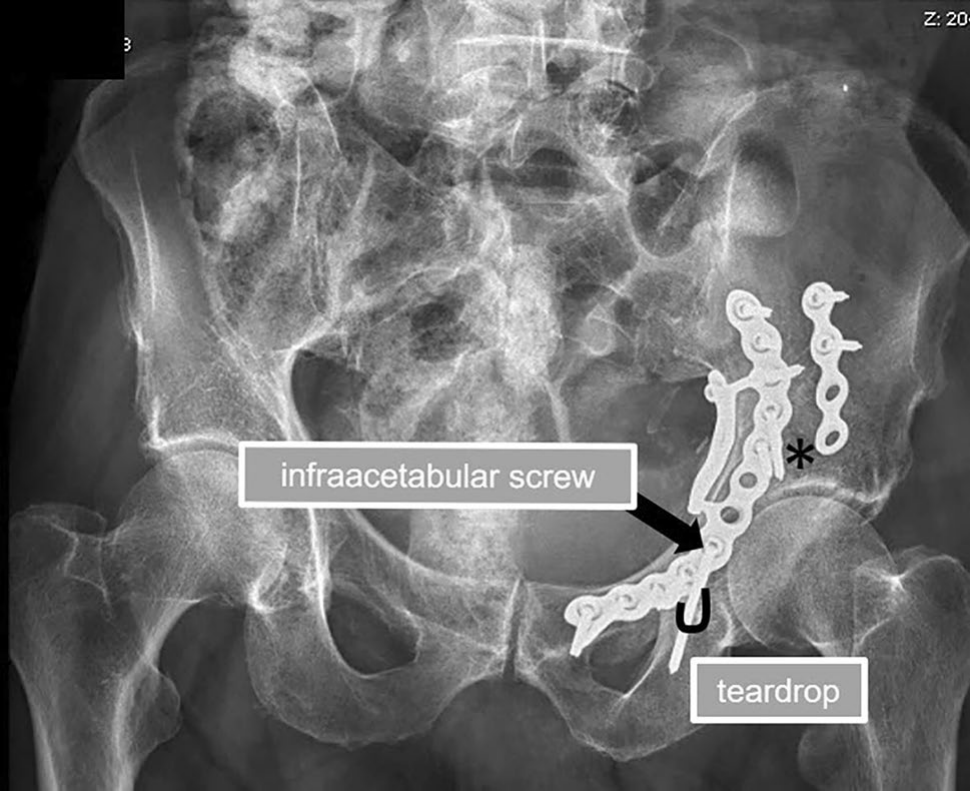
Postoperative anteroposterior radiograph of the pelvis showing an anatomic reduction (asterix) of the acetabular fracture (incomplete anterior column posterior hemitransverse) with break-out of the quadrilateral plate and impaction at the acetabular roof in a 79-year-old male patient. To enhance the fixation strength, an infra-acetabular screw (black arrow) was applied with the teardrop being the isthmus of the screw
